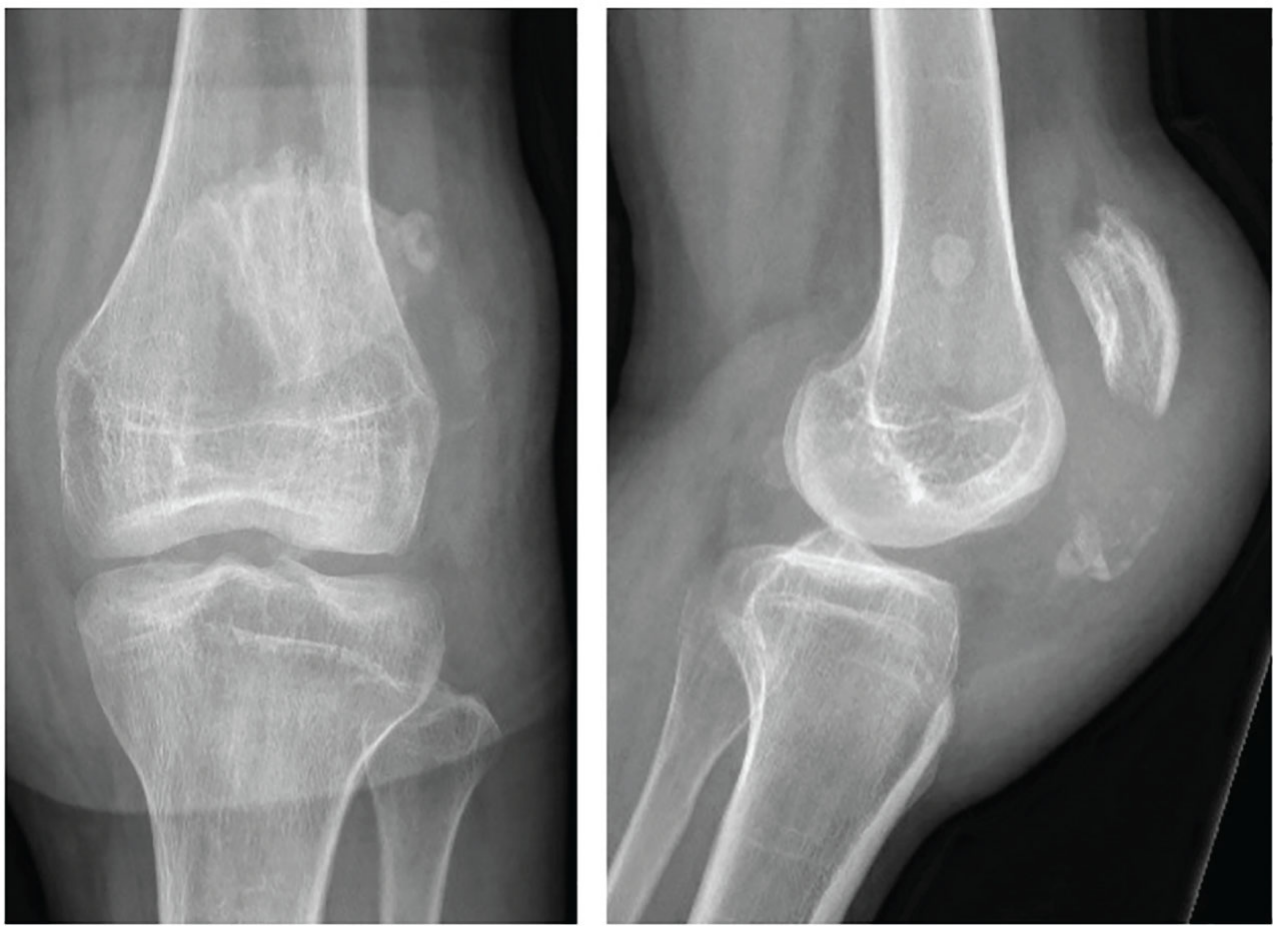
AP and lateral radiographs of the left patella showing an avulsion fracture of the inferior patella pole.
